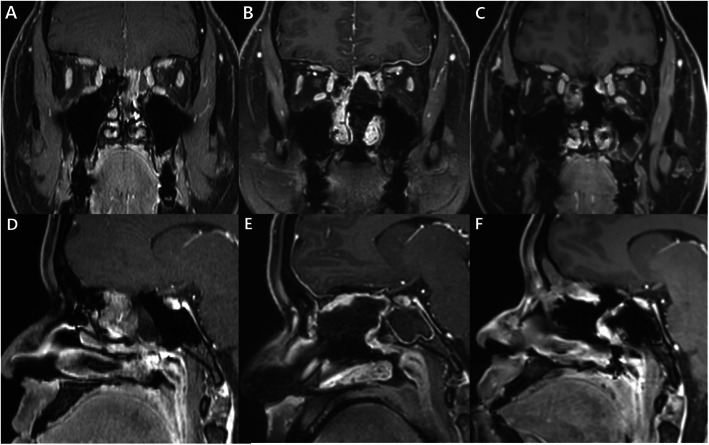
Images from Case 2. An olfactory neuroblastoma. a Enhanced coronal MRI reveals a mass with irregular margins on the left side of the upper nasal cavity extending to the frontal base. d The tumor can be seen invading the frontal base and extending to the anterior wall of the sphenoid sinus. b, e A well-enhanced PNSF and coverage state of the ASB defect can be seen in the postoperative coronal and sagittal MRI. c, f The follow-up coronal and sagittal MRI revealed stable disease status and well-healing state of the nasal mucosa 6 months after the operation. ASB: anterior skull base, PNSF: pedicled nasoseptal flap
